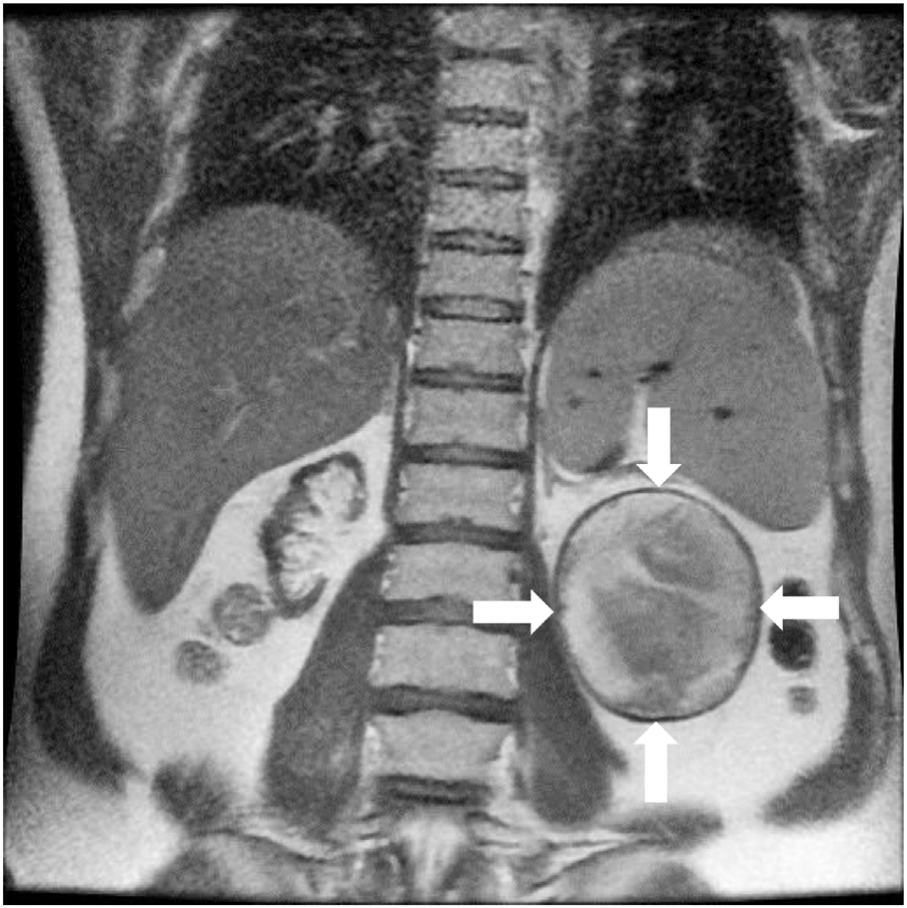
Image of the initially unclear cystic mass in the MRI scan of the abdomen.
